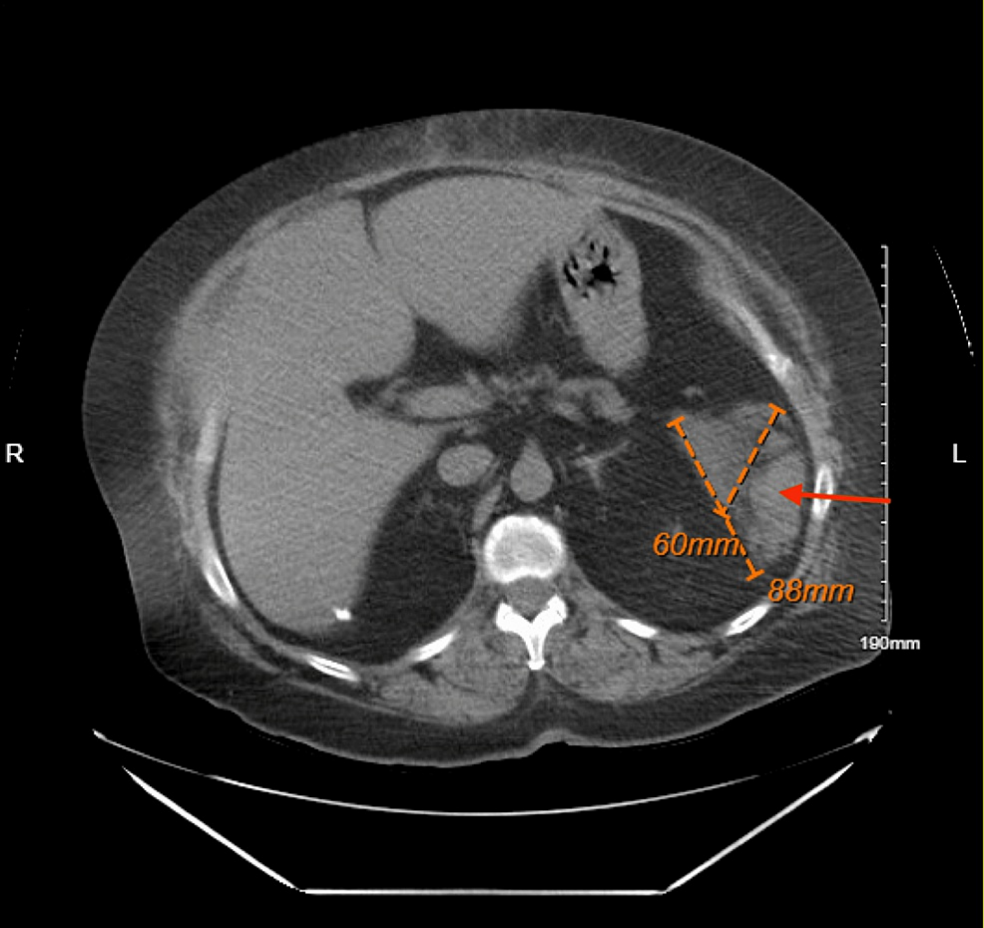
Computed tomography from a year prior to the patient's most recent visit depicting pseudocyst collection on the left side (red arrow) measuring 8.8 cm x 6.0 cm or 88 mm x 60 mm cm: centimeters; mm: millimeters
